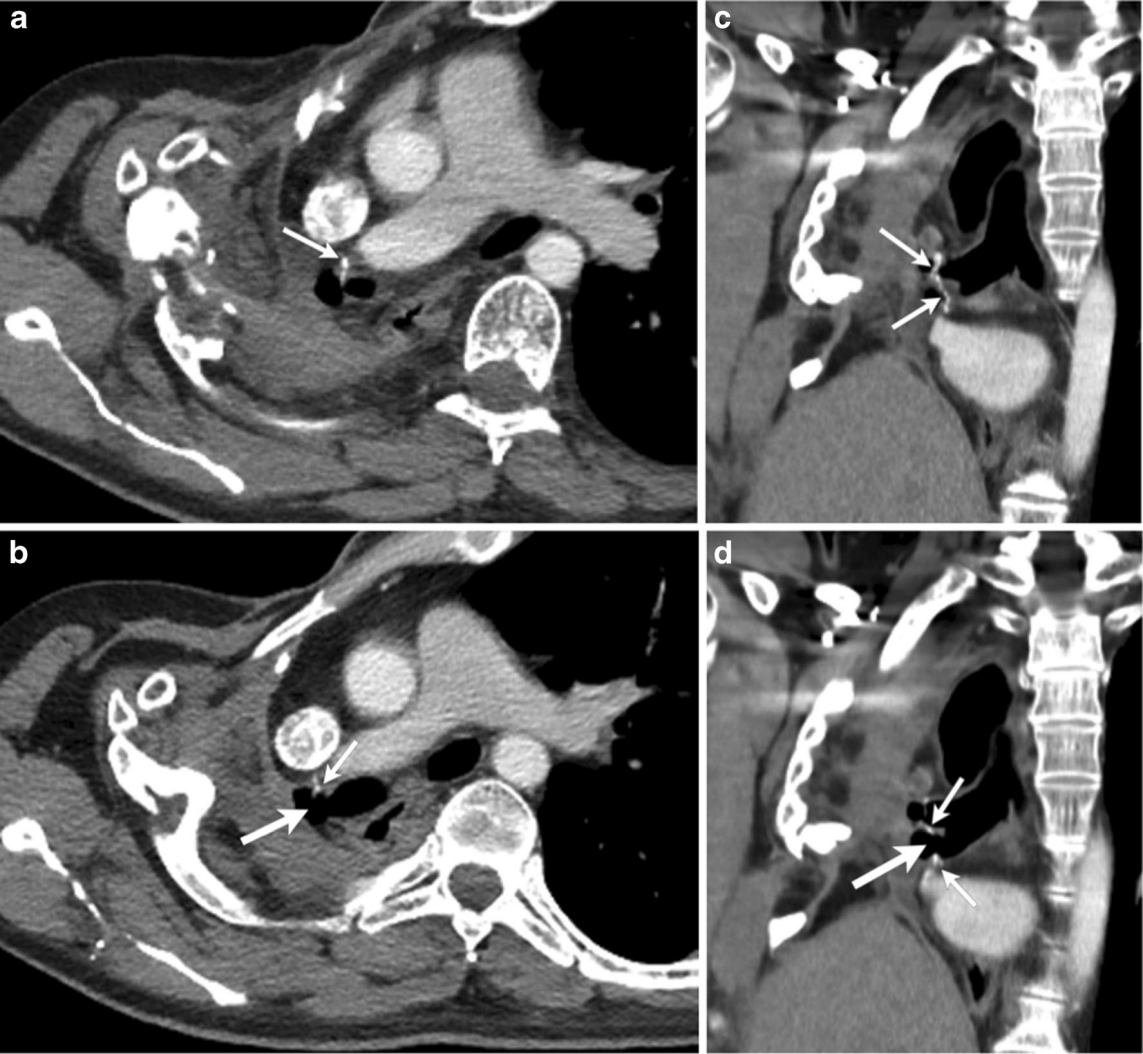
34-year-old male with history of gunshot injury; presenting with persistent pneumothorax post pneumonectomy. Axial (a,b) and coronal (c,d) post pneumonectomy CT images demonstrate a dehiscent bronchial stump communicating with the pleural space (*thick arrow*). Notice discontinuity of the suture line (*small arrows*)
